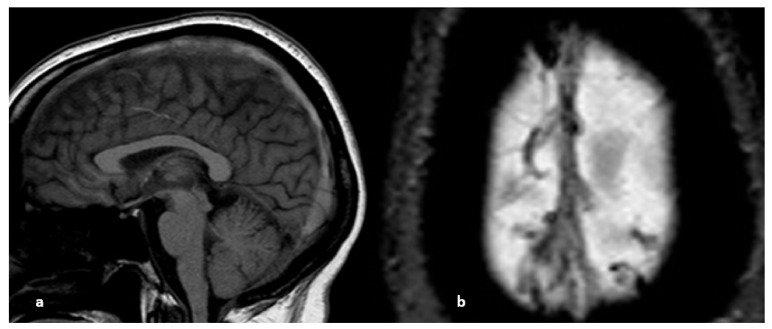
(a) Nonenhanced T1-weighted images, sagittal plane. Spontaneous hypersignal of the superior sagittal sinus suggests thrombosis. (b) T2* images, axial plane. Spontaneous hyposignal of the cortical veins of the vertex suggests cortical vein thrombi.
